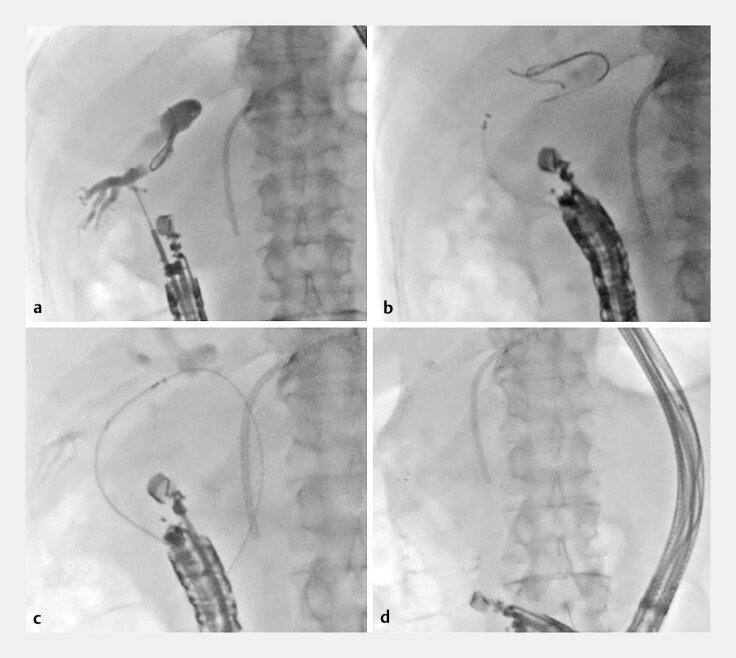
Endoscopic ultrasound-guided hepaticoduodenostomy.
